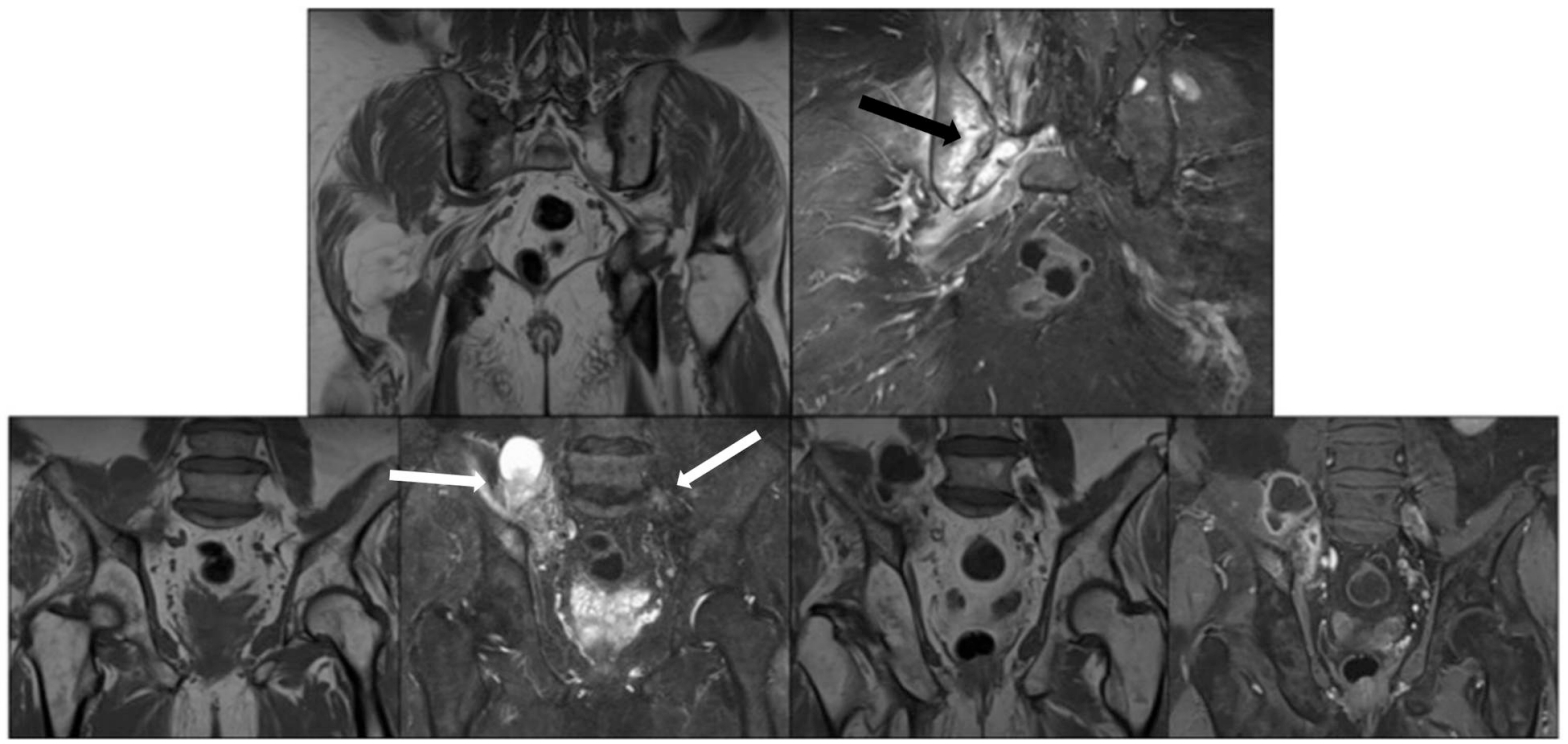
SI MRI evaluation during hospitalization with significant soft tissue extension. Upper line: T1-weighted sequence (on the left) and T2-weighted sequence (on the right) in the coronal section focused on the SI joints. Large sacroiliitis with extensive bone oedema (black arrow). Lower line from left to right: T1-weighted sequence, T2-weighted sequence, T1-weighted water sequence without gadolinium injection and T1-weighted water sequence with gadolinium injection showing abscesses in adjacent soft tissues (white arrows)
